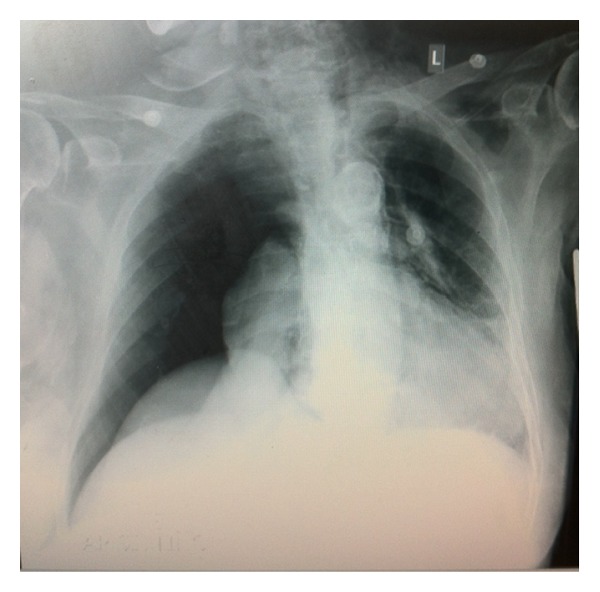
Chest X-ray showing right-sided pneumothorax. There is no intraperitoneal free air.
